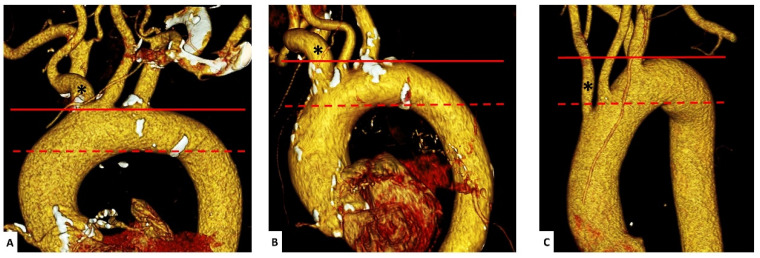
Types of the aortic arch. Pre-TAVI CTA in Volume Rendering Technique (VRT) for evaluation of the aortic arch. The types were defined as I (origin of the brachiocephalic artery at the height of the maximum cranial curvature of the aortic arch; (A), Type II (origin between the maximum cranial and caudal curvature; (B) and Type III (origin below the caudal curvature; (C). The maximum cranial (-----) and maximum caudal (-) curvature of the aortic arch as well as the brachiocephalic artery (*) are delineated.
